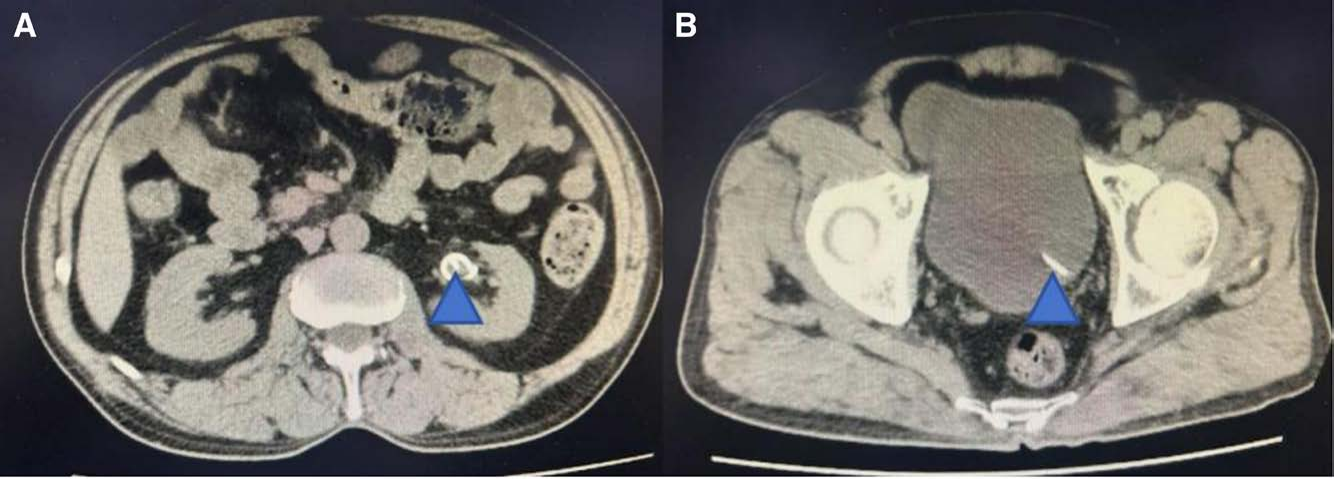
Abdominal computed tomography. (A) The ureteral stent is located in the renal pelvis (indicated by triangular star). (B) The ureteral stent is located in the bladder (indicated by triangular star).
